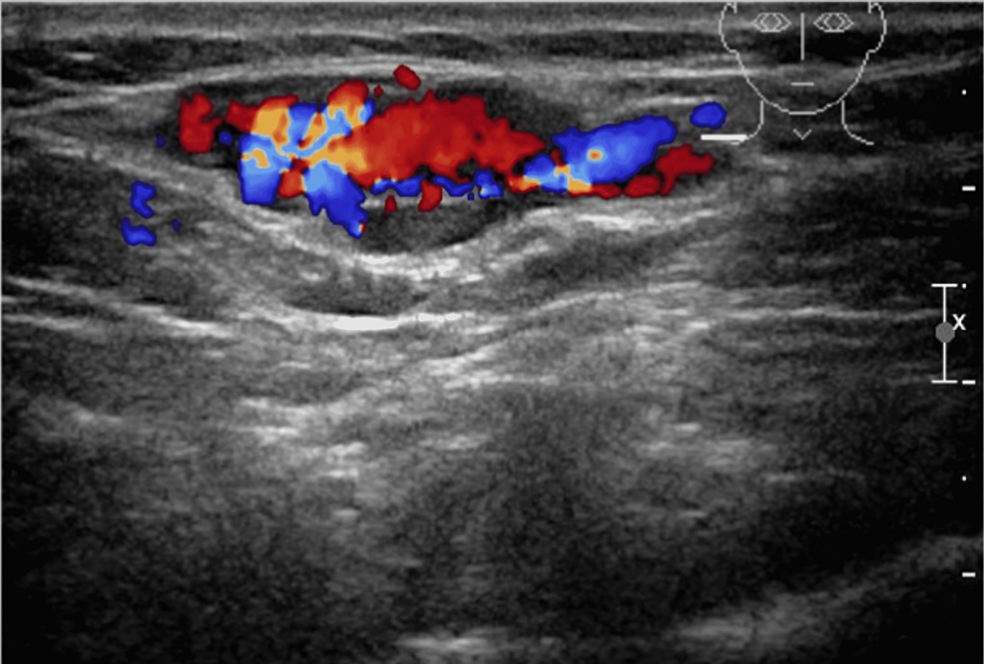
Ultrasound of the neck with color Doppler revealed a vascular structure containing turbulent blood flow. It measured 2.9 x 1.2 cm and appeared partially thrombosed with communication with the venous system.
